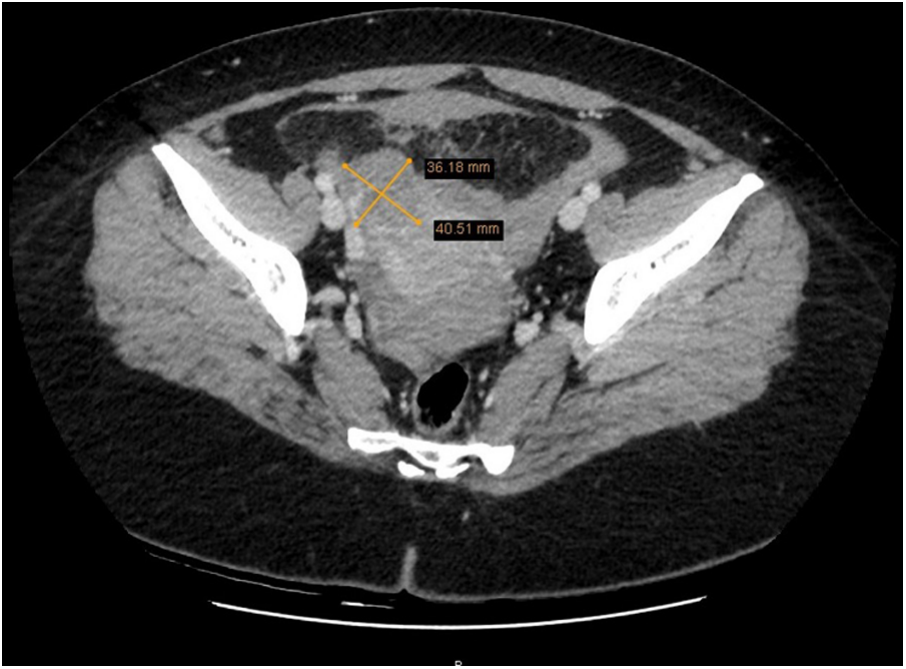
Abdominal and pelvic computed tomography showing a 35 mm heterogeneous vascularized formation in the projection of the right ovary.
